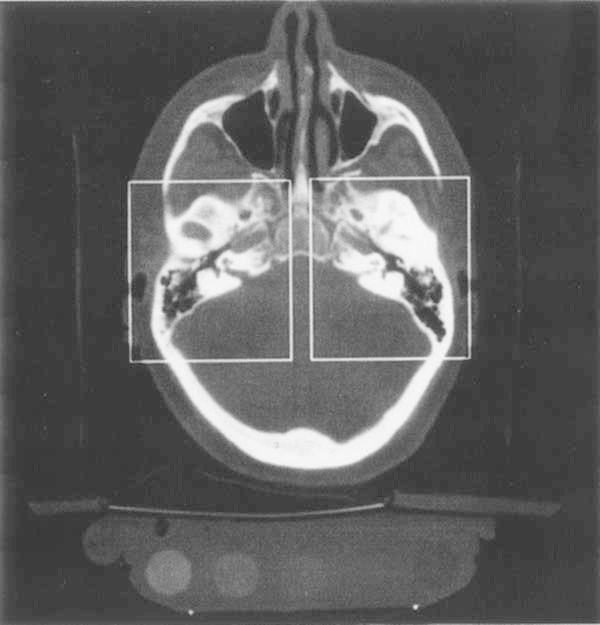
Axial quantitative computed tomography scan image of the head showing the calibration device beneath the head. White squares outline the subregions sampled (128 × 128 pixels). 16 Reprinted from *Hearing Research*, Vol 83, Monsell E, Cody D, Bone H, et al., Hearing loss in Paget's disease of bone: the relationship between pure-tone thresholds and mineral density of the cochlear capsule, Pages 114–120, Copyright 1995, with permission from Elsevier.
